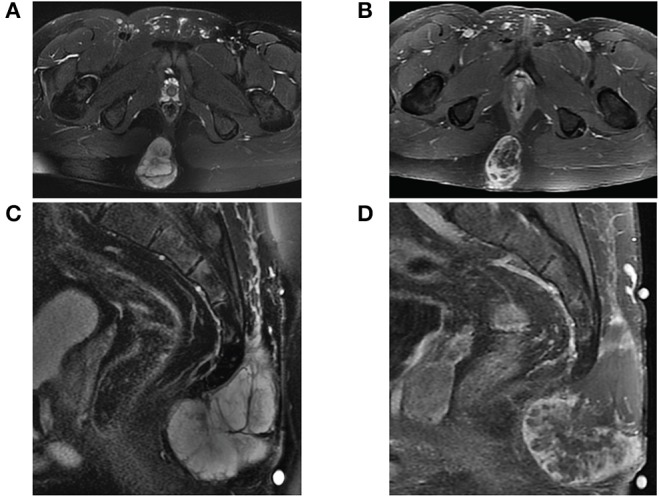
MRI of sacrococcygeal mass prior to initial resection. (A) Axial view of fat-saturated T2-weighted and (B) contrast-enhanced T1-weighted sequences demonstrate a lobulated expansile mass confined to the soft tissues affixed between the gluteal muscles. The mass on sagittal view is (C) T2 hyperintense well-encapsulated within the post-sacral soft tissues without invasion into the sacrococcygeal space and (D) heterogeneously enhancing with central necrosis.
